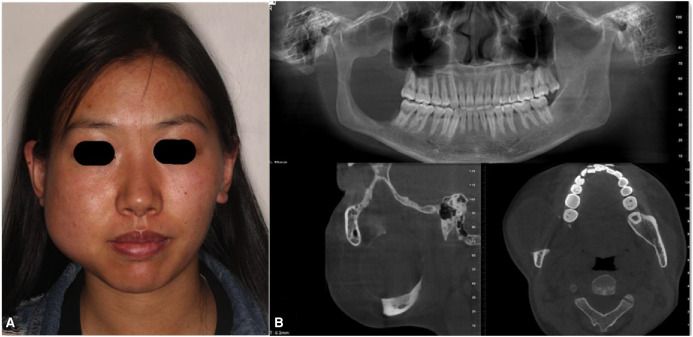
Clinical examination revealing a significant bulge of the right mandibular: cone beam computerized tomography (CBCT) demonstrating that (A) there was a bone mineral density reducing area of nabout 4.8 cm × 4.6 cm × 4.9 cm in the right mandible from 47 to the mandibular ramus region and (B) Buccolingual bulge was obvious.
